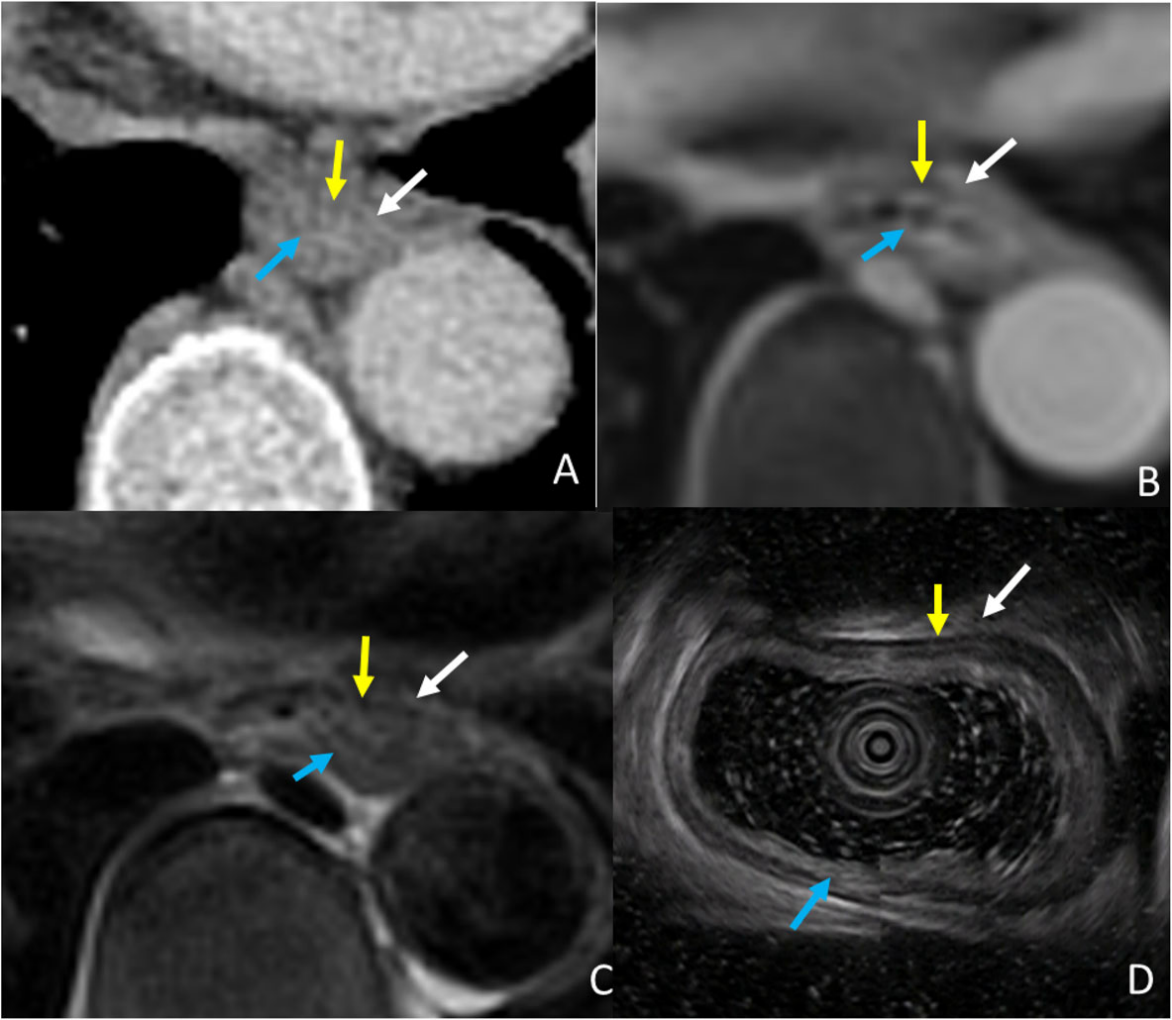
Normal esophagus of a 52-year-old man, demonstrating muscularis mucosae (blue arrow), muscularis propria (yellow arrow) and adventitia (white arrow) in CT (A), 3D-GRE, (B) T2WI-msTSE (C), and EUS (D)
